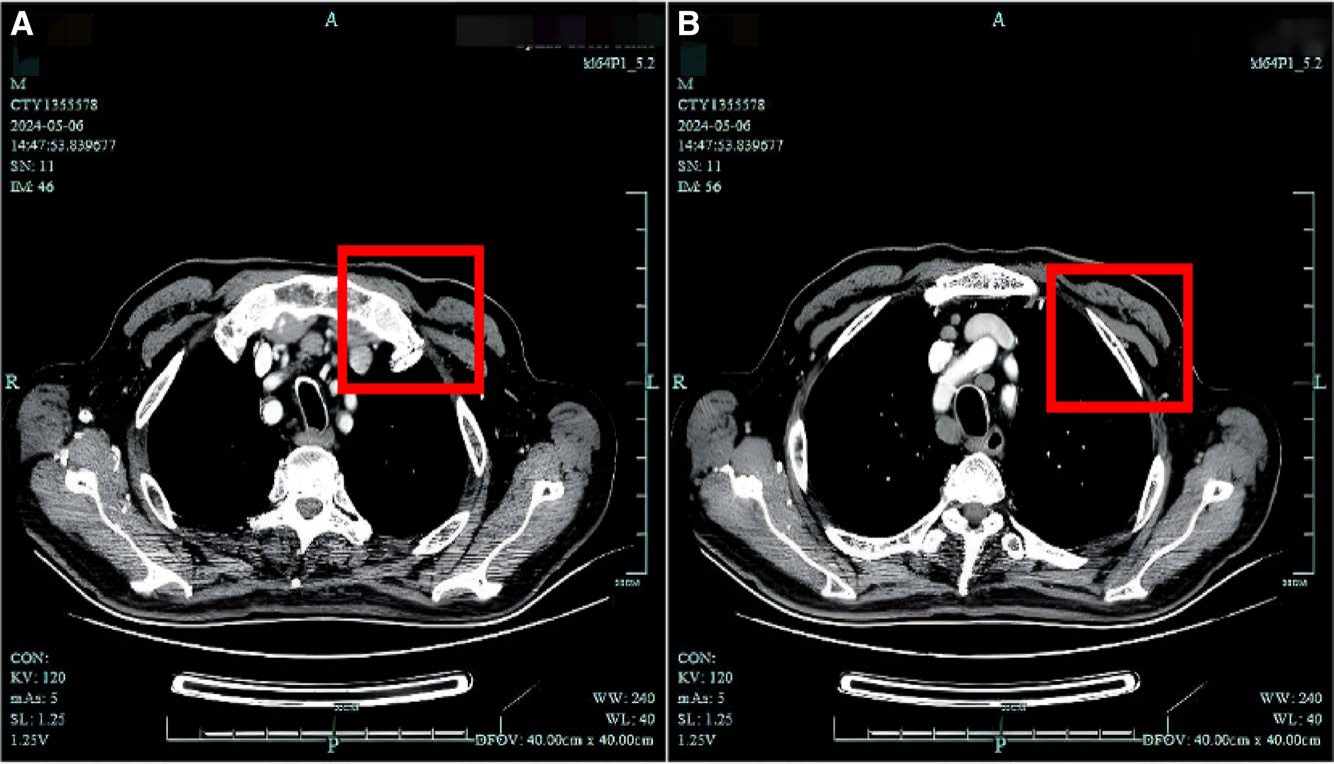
(A) and (B) Chest CT showing mediastinal lymph node abnormalities. CT = computed tomography.
